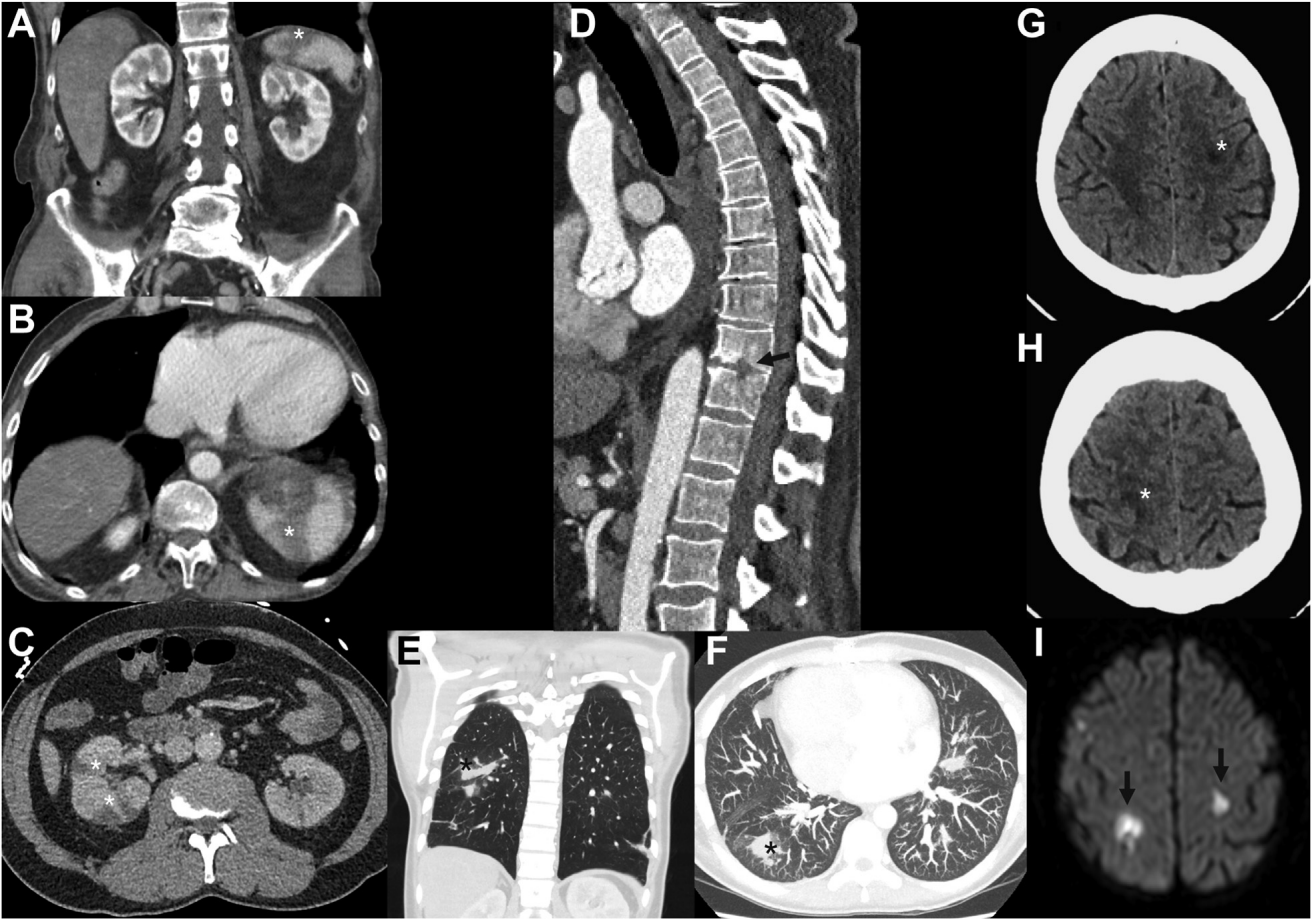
Extracardiac complications shown on computed tomography. Wedge-shaped hypoattenuating regions in the spleen and kidney (A, B, and C) are compatible with infarct due to embolized vegetations. Destruction of part of the vertebral bodies due to spondylodiscitis (D) and pulmonary septic emboli (E and F). Cerebral ischemia due to septic emboli seen on computed tomography (G and H) and magnetic resonance imaging (I).
